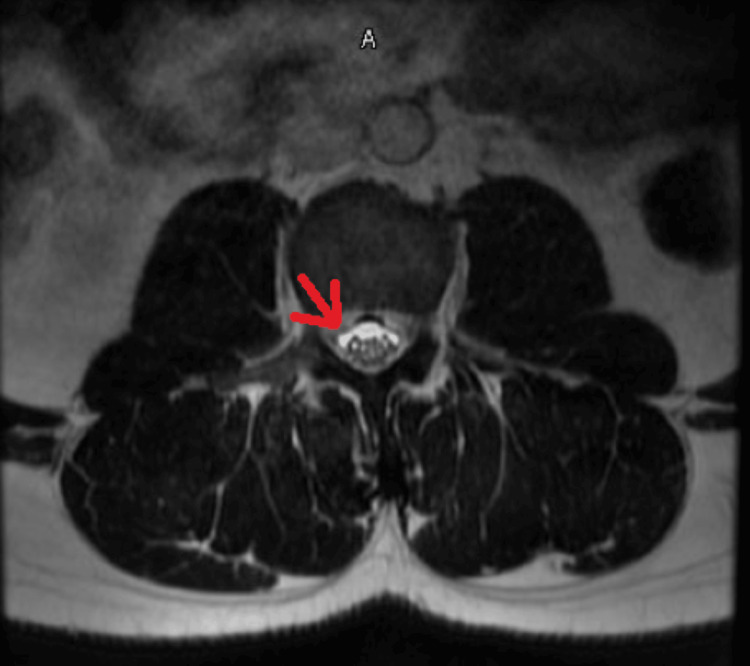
Spine MRI (T1 weighted post contrast with fat suppression) showing symmetrical bilateral nerve root enhancement (arrow). A in the figure represents axial plane.
